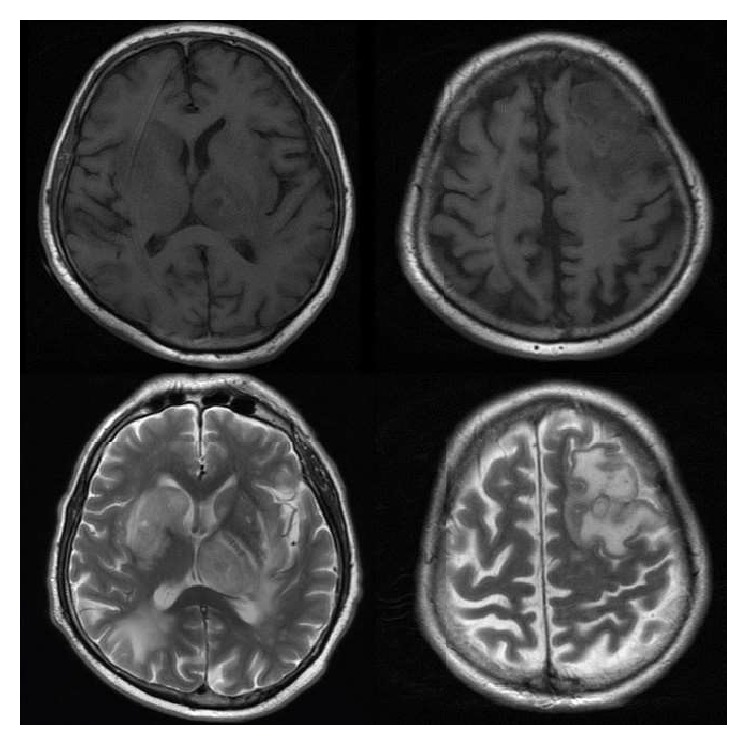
MRI at admission (day 34). Top two images are T2-weighted, middle two are T1-weighted, and bottom two are T2/FLAIR-weighted. Multifocal enhancing lesions in both cerebral hemispheres and in the left cerebellum concerning metastasis, infection, or inflammatory etiology. Redemonstration of areas of hemorrhage in the left frontal lobe is seen on CT scan. 4 mm left to right midline shift is present. Centrally necrotic lesion in the left thalamus measures 14 × 11 mm. Lesion in the right caudate head measures 11 × 10 mm. Enhancing lesion in the posterior left cerebellum measures 9 × 6 mm.
